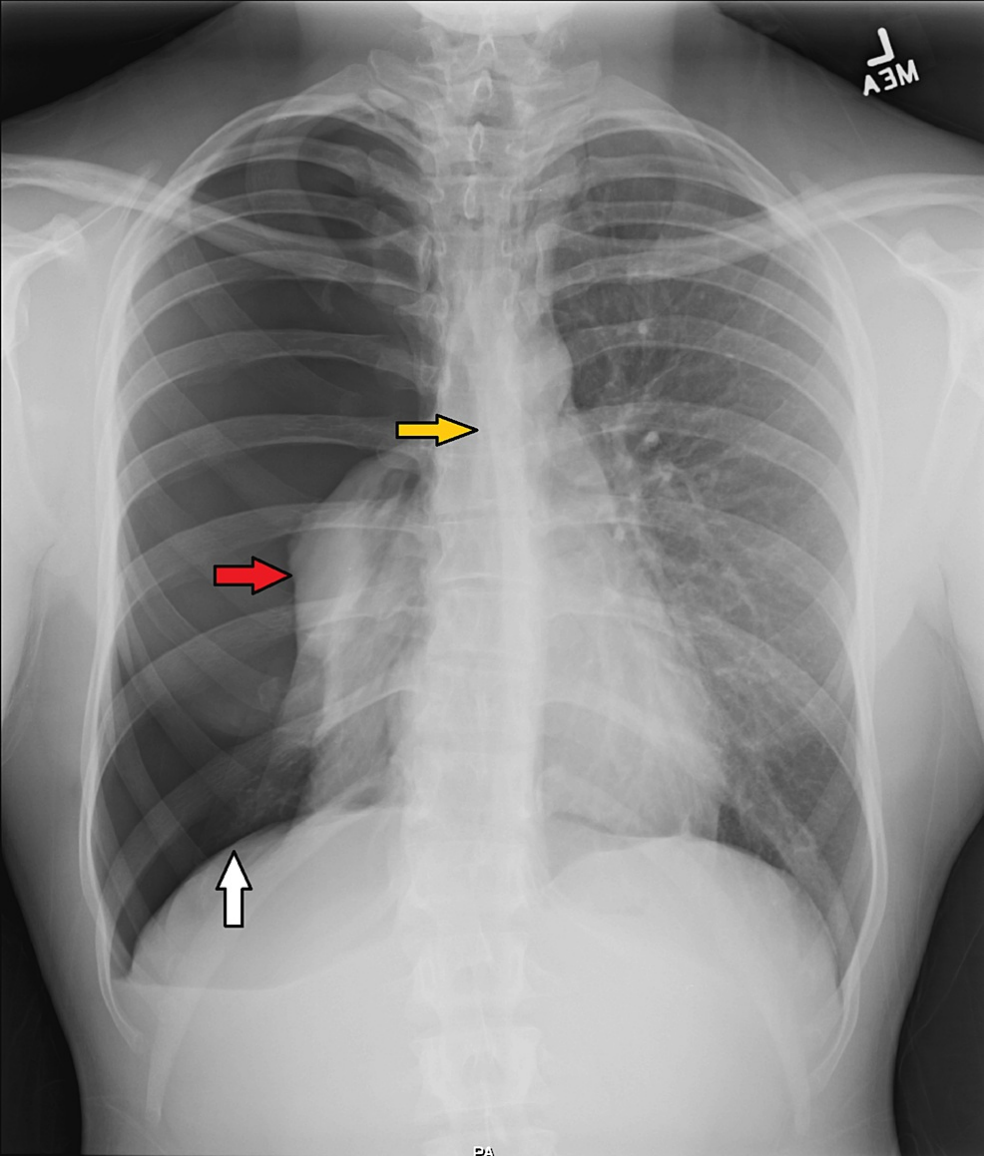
Chest X-ray showing complete right pneumothorax (red arrow). Developing mild mediastinal shift is noted (yellow arrow) with depression of the right hemidiaphragm suggesting tension (white arrow).
